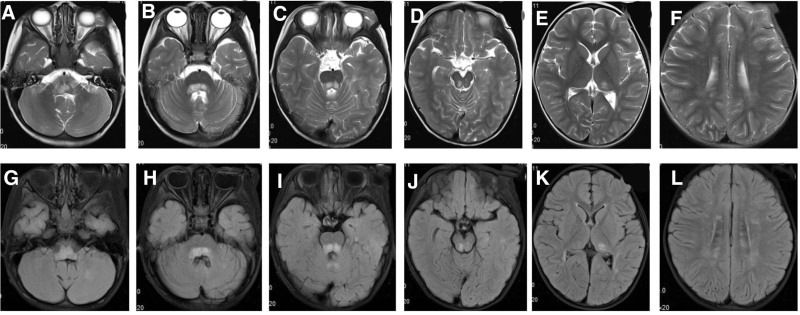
Cranial MRI of Case 1 at the age of 3 years and 2 months. Multiple patchy, slightly long T1, long T2, and FLAIR high signals found at white matters of bilateral frontal, parietal, and occipital lobes, as well as bilateral para-ventricular white matters, left thalamus, bilateral cerebellar hemispheres, and dorsal pons, with relatively clear boundaries are. Some lesions were small round shapes; some lesions of the left thalamus and dorsal pons had higher T2WI signals than before (March 24, 2021). The posterior parts of the bodies of bilateral lateral ventricles were slightly widened. FLAIR = fluid-attenuated inversion recovery, MRI = magnetic resonance imaging, T2WI = T2-weighted imaging.
